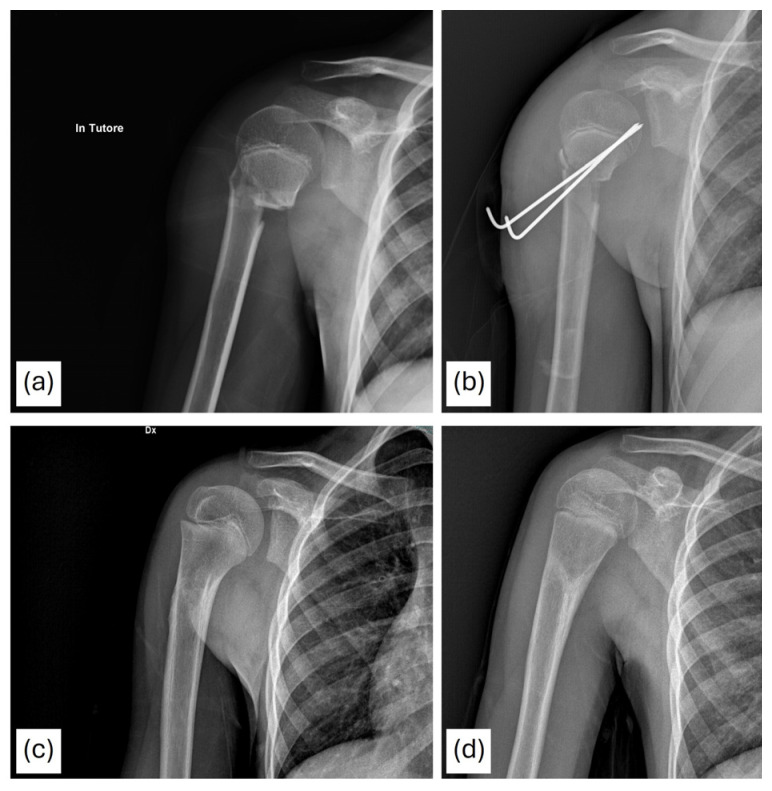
A 10-year-old patient with a Neer type IV fracture treated surgically by closed reduction and fixation with Kirschner wires. Postoperative radiographs are presented (a,b), along with a radiographic follow-up at 6 months (c,d).
